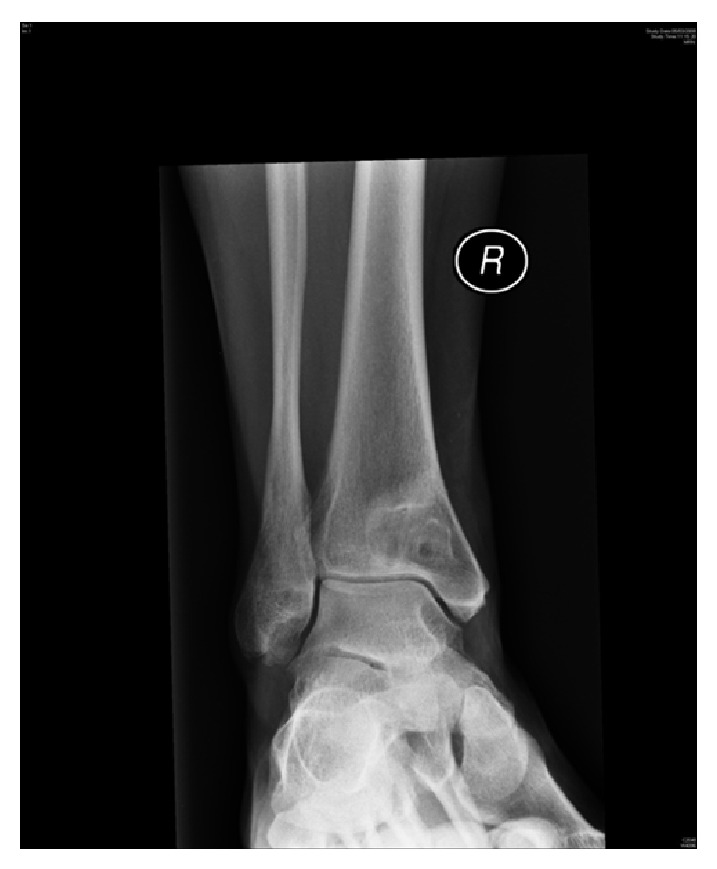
AP X-ray showing reoccurrence of cystic lesion distal tibia.
